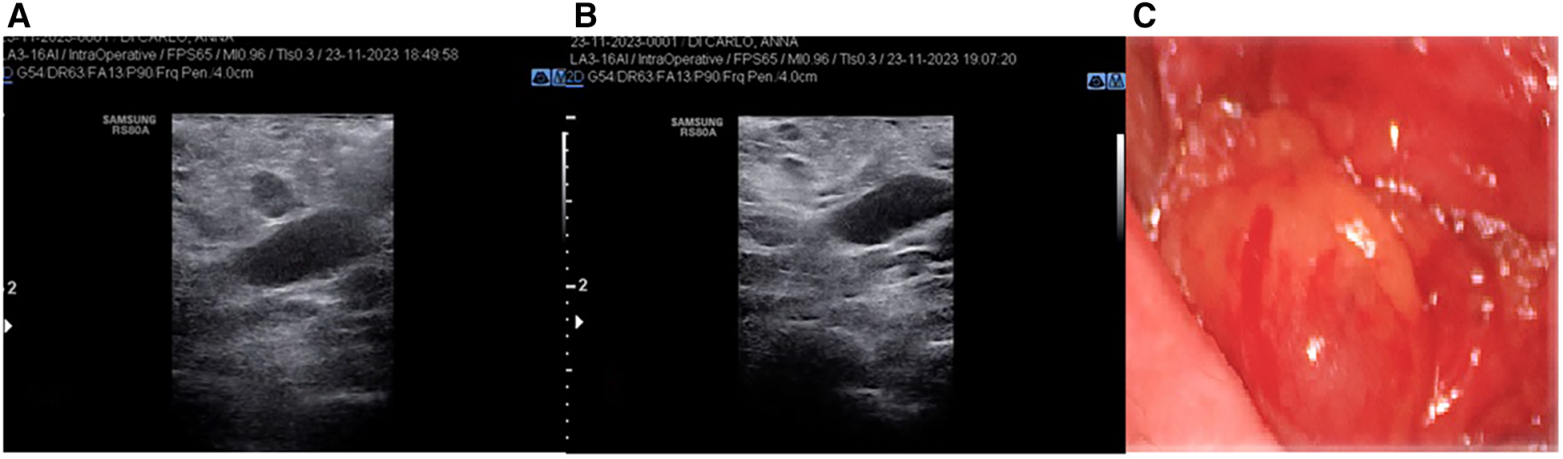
(A) Intraoperative ultrasound detection of suspicion node; (B) intraoperative ultrasound control after node's removal (C) pathological node.
